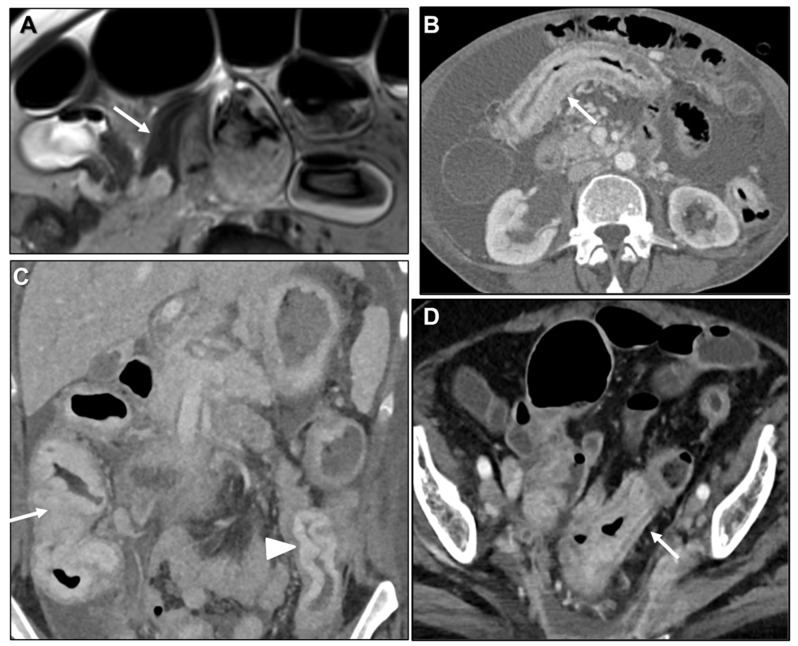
Different lengths of GI involvement. (A) MR image: Axial T2WI. Linitis plastica-like metastasis to small bowel (arrow) from invasive lobular carcinoma (ILC). (B) Axial contrast-enhanced CT (CE-CT). Linitis plastica-like metastasis to transverse colon (arrow) from ILC. (C) Coronal MPR CE-CT. Linitis plastica-like metastasis to ascending (arrow) and descending colon (arrowhead) from gastric adenocarcinoma. (D) Axial CE-CT. Linitis plastica-like metastasis to sigmoid colon (arrow) from ILC.
